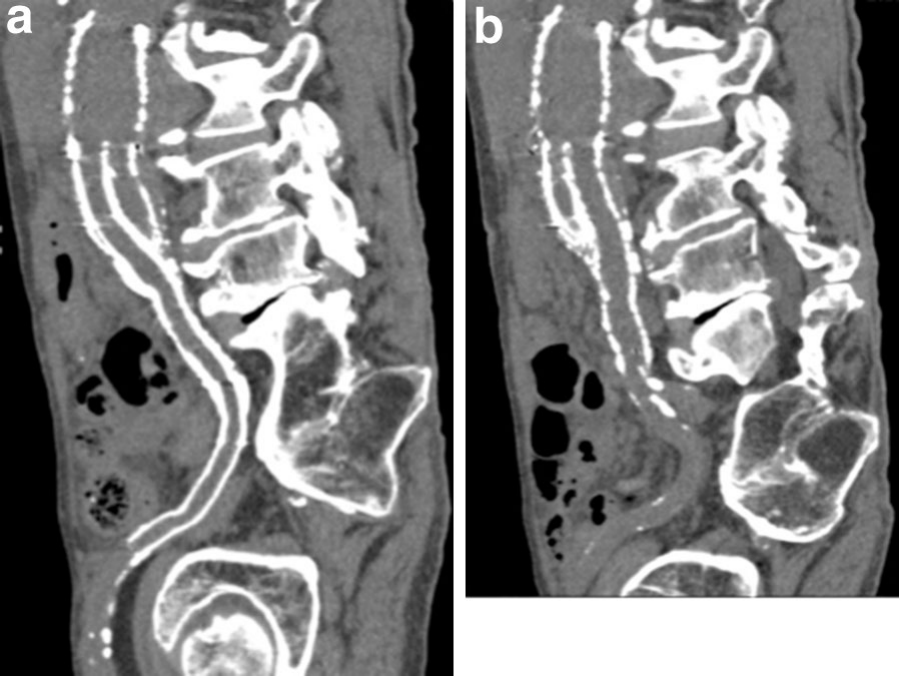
Computed tomography angiography findings. Good expansion of the two implanted stents was observed. No kink or stenosis was observed in either limb (a the left limb, b the right limb)
